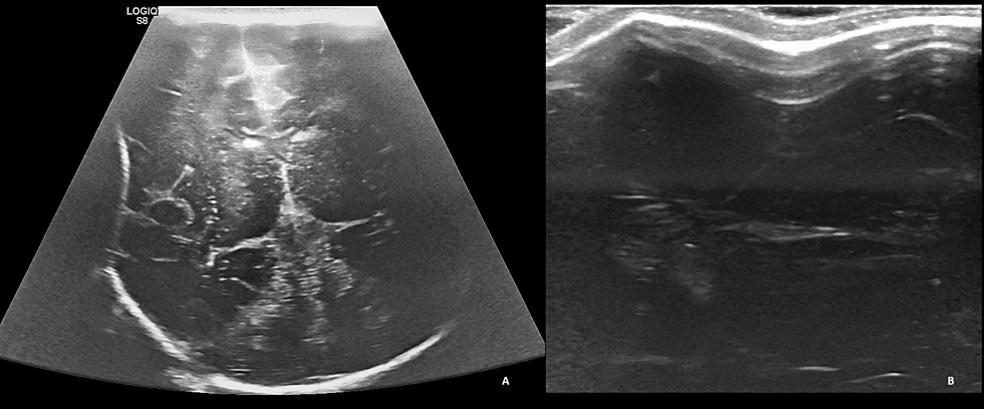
Cranial ultrasound of neonatal skull depression Performed on the third day of life and revealed a depression on the right parietal bone with no signs of fracture, intracranial structural anomalies, or bleeding (A-coronal view; B-M mode at the location of skull depression)
